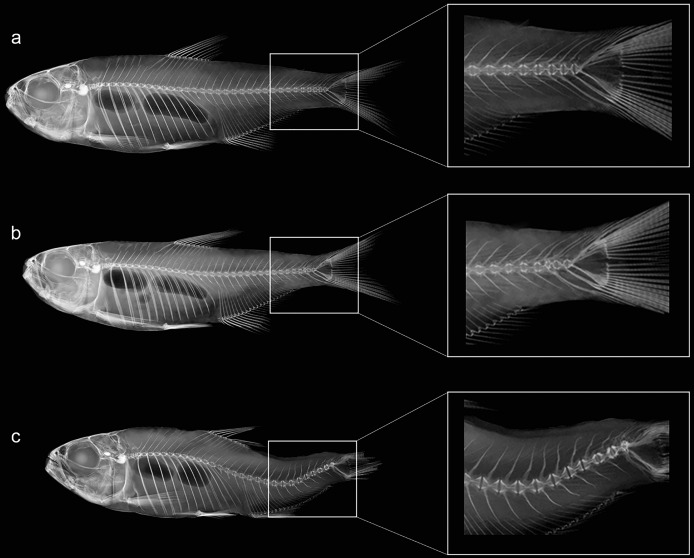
Anatomical patterns established for *Psalidodon* aff. *fasciatus* from *Furna 2* in VVSP, based on X-ray images of spine morphology. (A) Normal pattern (Np)–no deformation; (B) moderate malformation (Mm)–minimal spine deviation; (C) severe malformation (Sm)–high spine deviation.
